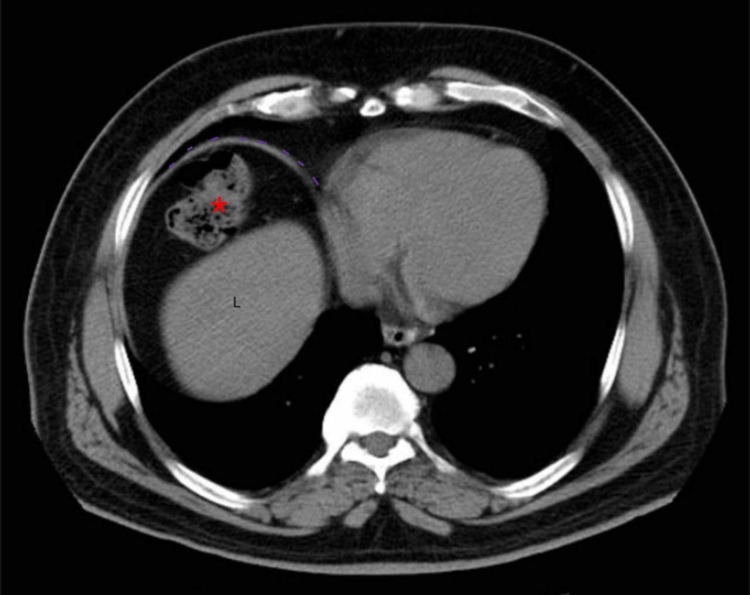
Axial non-contrast computed tomography (CT) showing interposition of the large intestine (*) in between the liver (L) and diaphragm (dotted lines)
